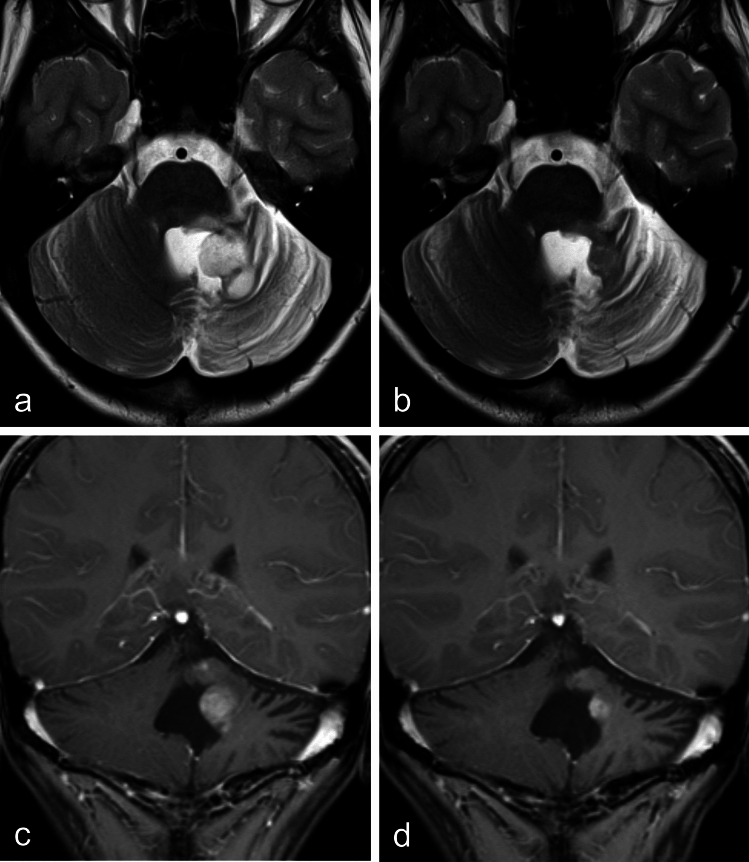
Case of partial response. A 7-year-old girl was diagnosed with a pilocytic astrocytoma. a Following incomplete resection, local progression was observed four years later. The residual tumor appeared hyperintense on T2-weighted images compared to the parenchyma of the unaffected right cerebellar hemisphere. Conventional photon radiotherapy was then initiated. b Two years after radiotherapy, both the tumor size and the T2 signal intensity had significantly decreased. c Contrast-enhancing tumor portions on baseline MRI were still present two years post-therapy (d)
